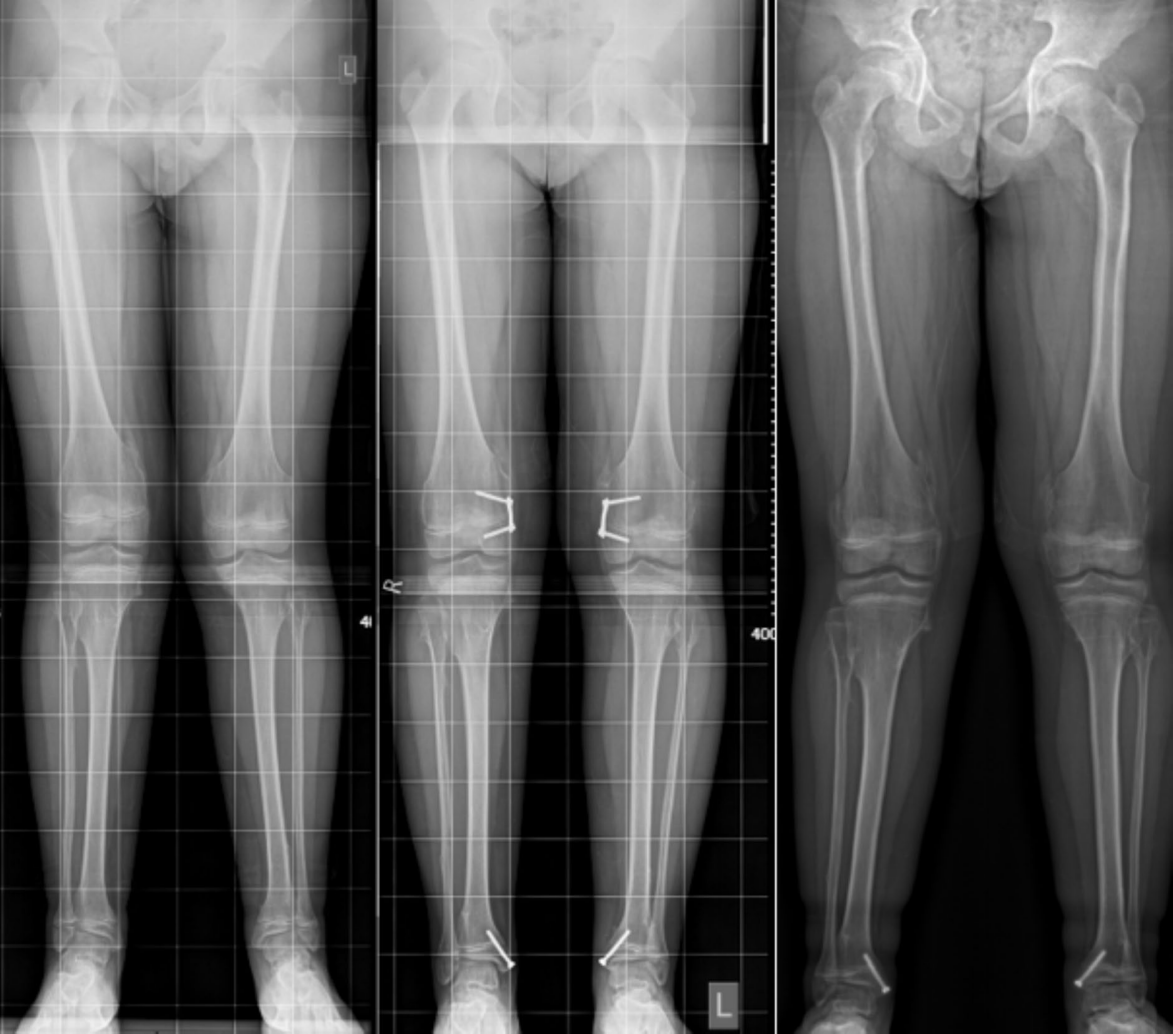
Radiographs of a patient pre-, during and post-coronal plane angular deformity correction
